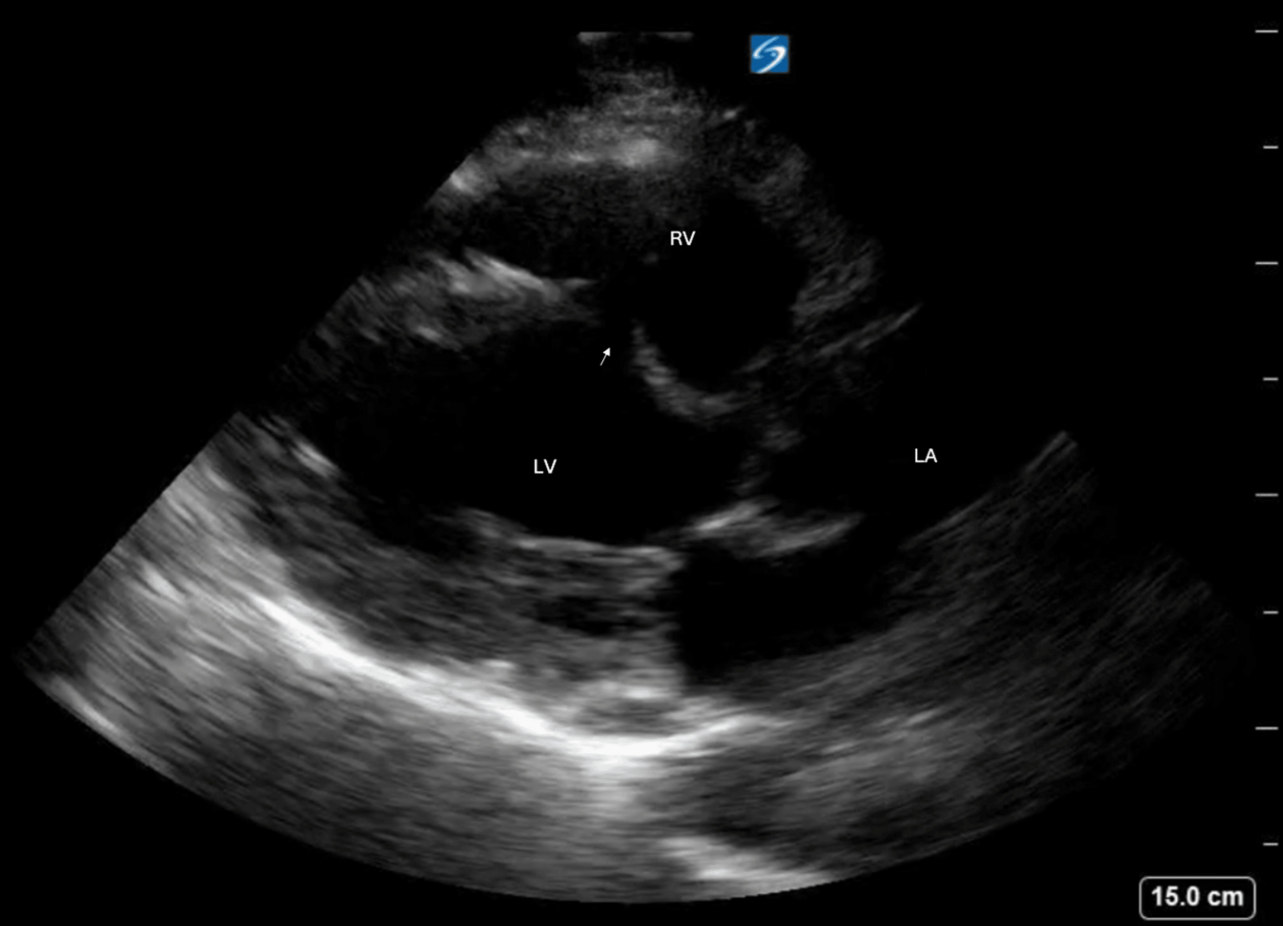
Parasternal long axis view with rupture of ventricular septum with arrow indicating VSR VSR: Ventricular septal rupture
